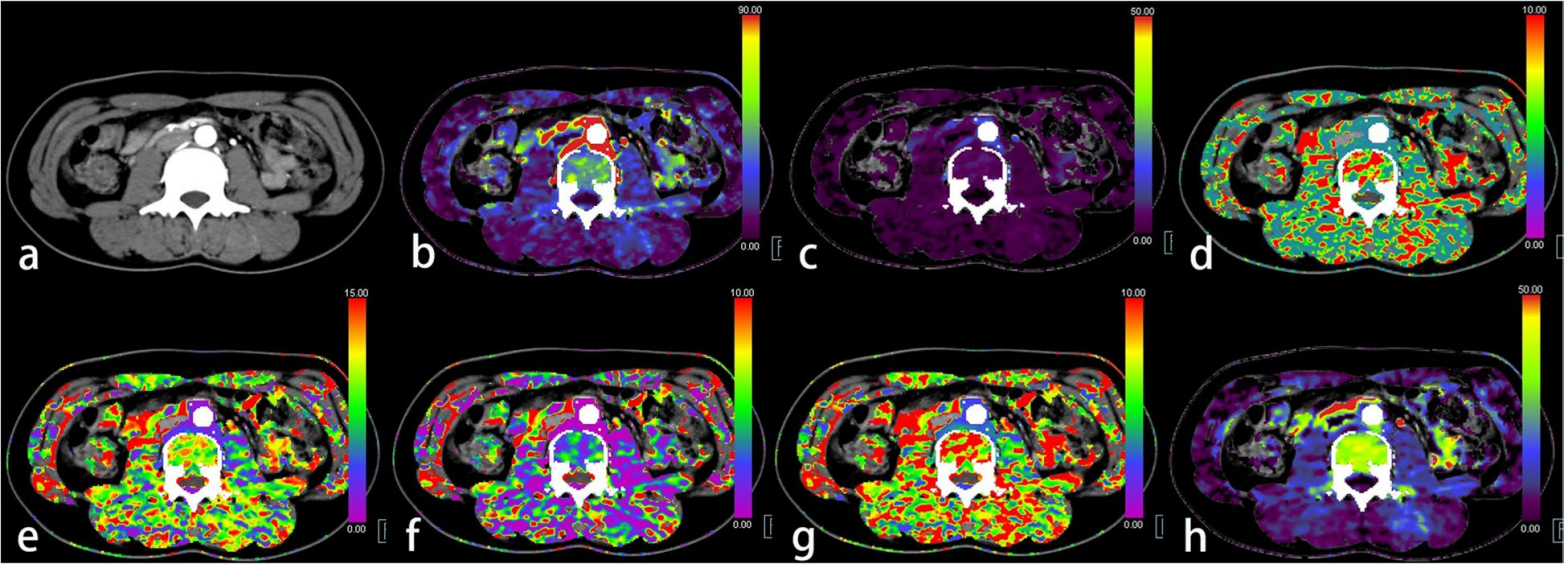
A 25-year-old female with normal bone mineral density, normal muscular mass, and low-grade sigmoid colon cancer (T_3_N_0_M_0_). a Axial abdominal contrast-enhanced CT image located at the central level of the vertebral body of lumbar 3. b The blood flow (BF) map of the CT perfusion (CTP). c The blood volume (BV) map. d The mean transit time (MTT) map. e The time to drain (TTD) map. f The time to start (TTS) map. g The time to maximum (T_max_) map. h The flow extraction product (FE) map
